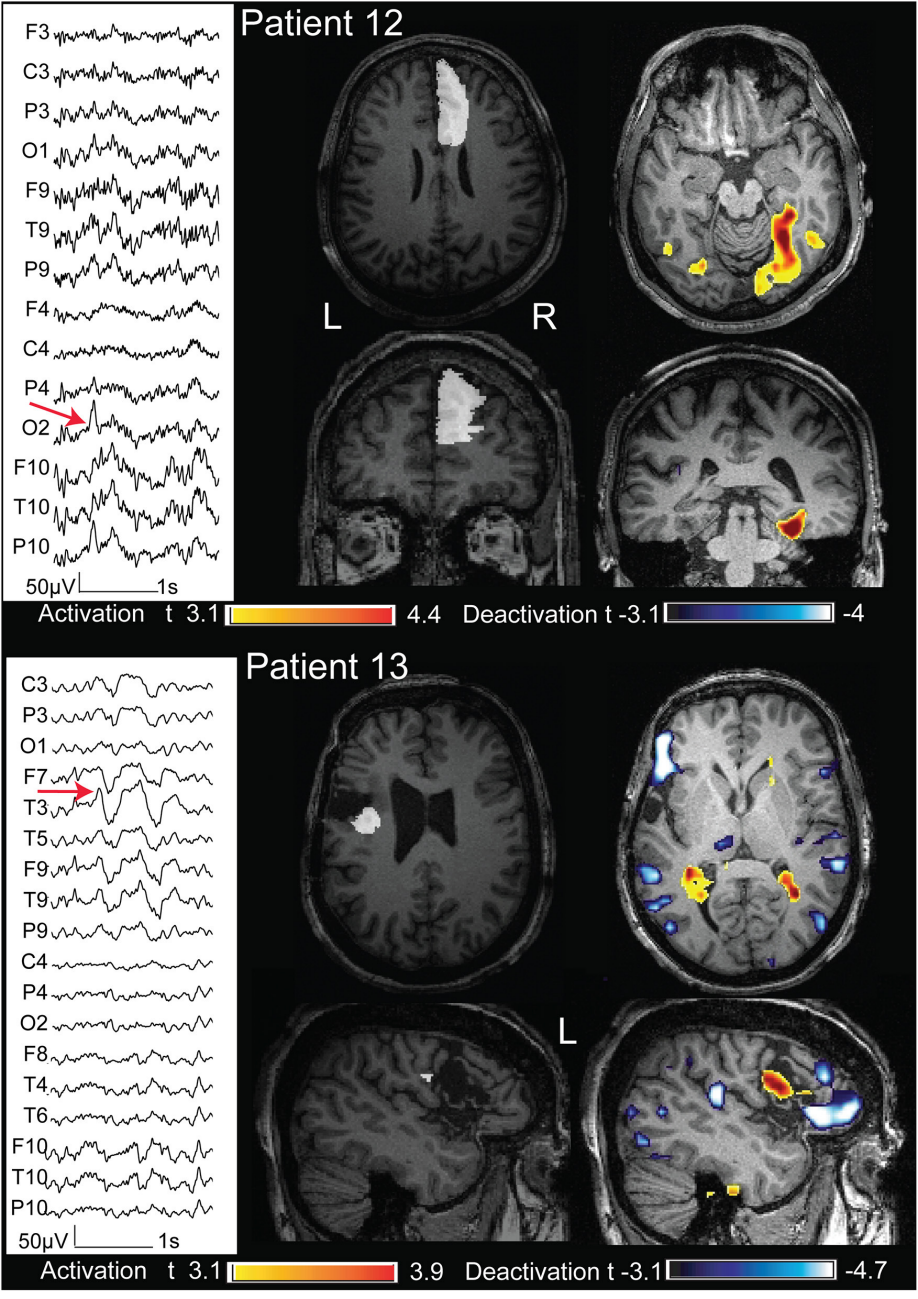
Above: case 12. The marked events were spikes max at P10 O2 (the electrode T6 had an artifact during the acquisition). The BOLD response showed an activation with max t value in the right fusiform gyrus. The removed cortex cointaining the FCD was the right supplementary motor area (marked in white). Below: case 13. The marked events were T3 spikes. This patient had three operations and the surgical bed is evident on the structural MRI. The cortex removed during the last operation (marked in white) showed the presence of a FCD. The BOLD response showed a max deactivation more anterior than the removed cortex. These 2 cases are not concordant.
